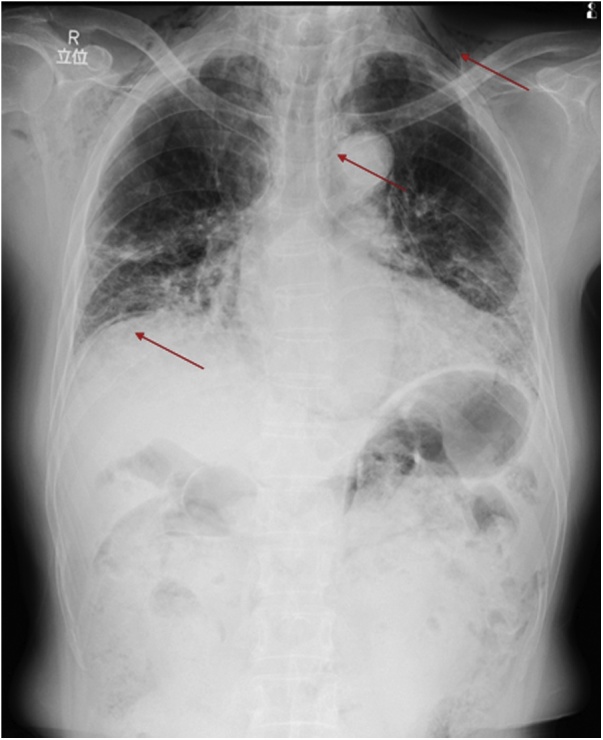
Chest radiography showing subcutaneous emphysema of the neck, mediastinal emphysema, and subdiaphragmatic free air (red arrows).
